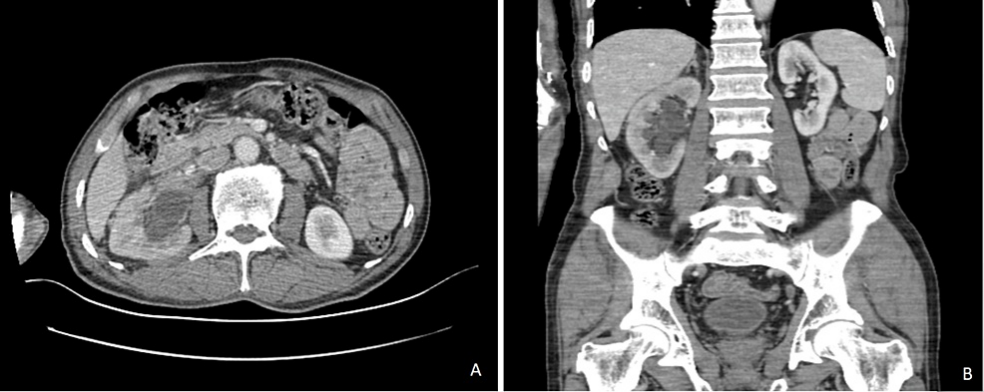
CT scan showing a spiculated contoured mass in the right kidney. (A) Axial plana, (B) coronal plane
